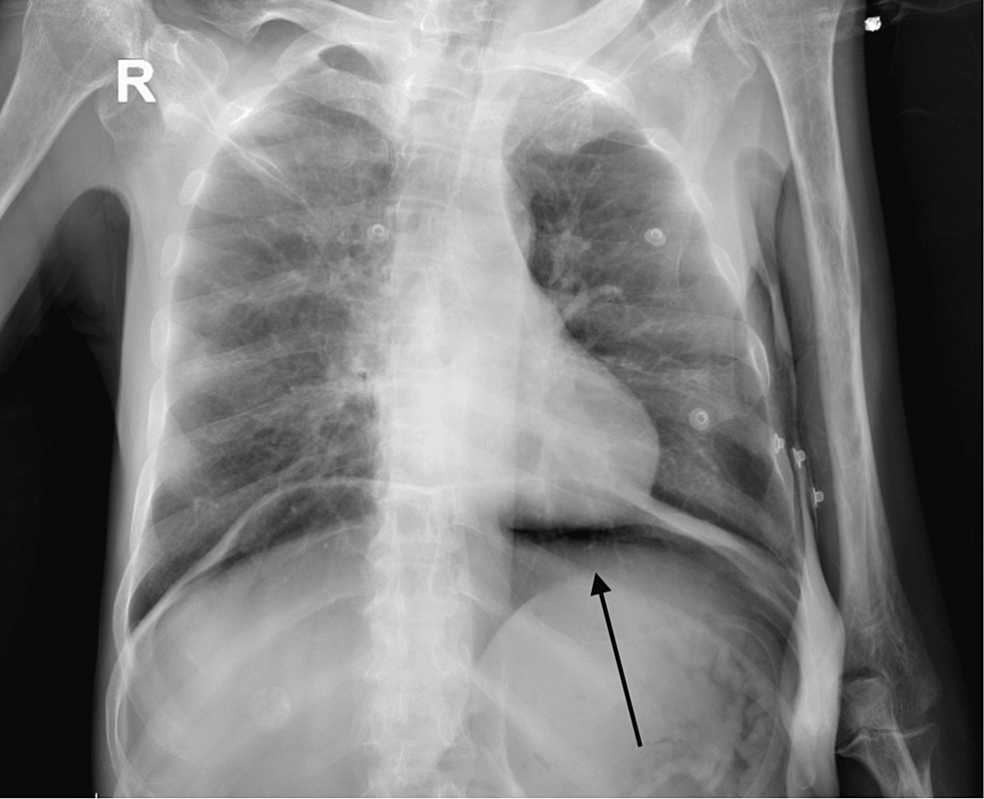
Chest X-ray of the patient on emergency room arrival.
